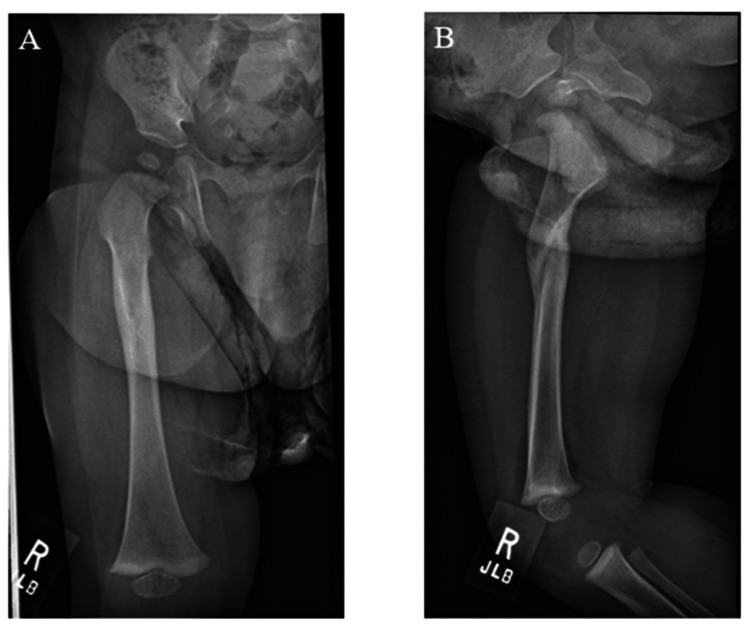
Final imaging for case 1, including (A) AP and (B) lateral radiographs of the right lower extremity demonstrating the healing of the proximal femoral diaphyseal fracture
